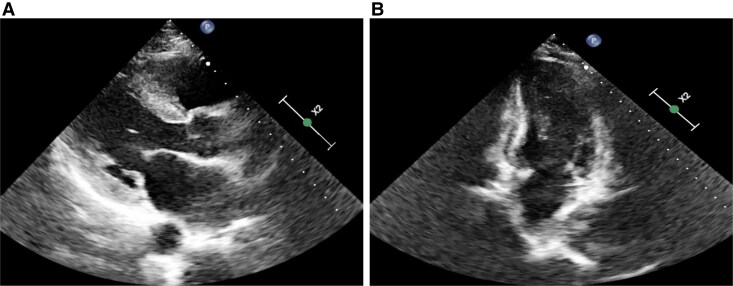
Transthoracic echocardiography demonstrating subtle hypokinesia of the apical septal and mid anterolateral segments, in the setting of mild left ventricular hypertrophy with preserved global systolic function. (*A*) Parasternal long-axis view. (*B*) Apical two-chamber view.
